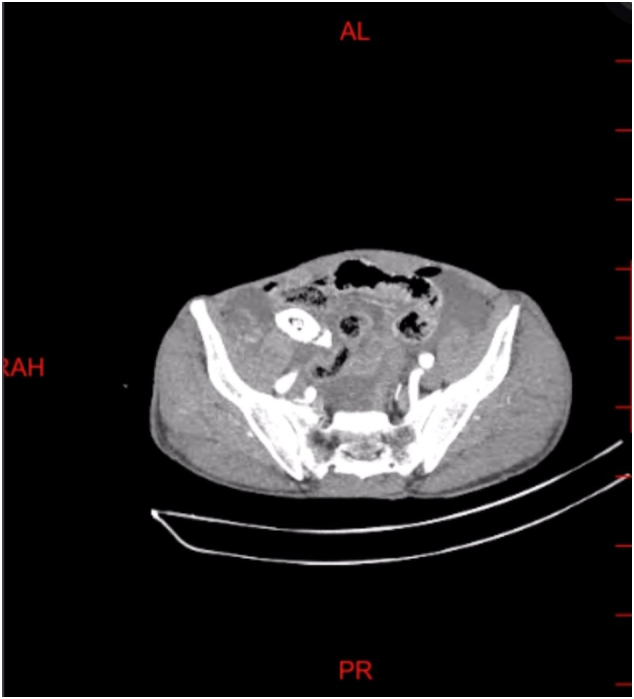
Axial view of abdominopelvic CT-scan with IV contrast.
